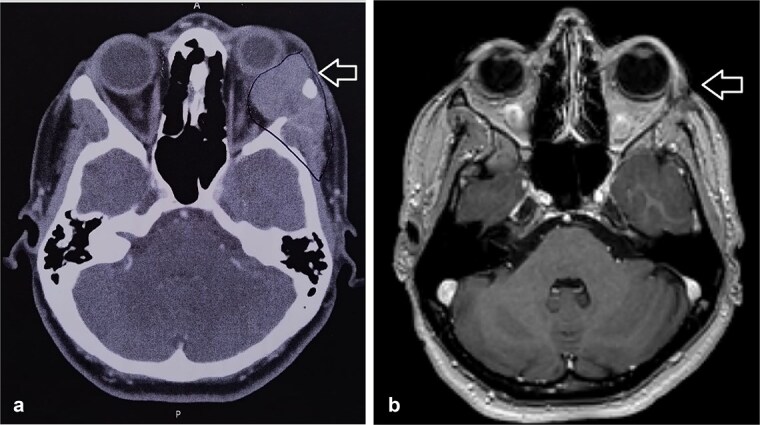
(a) CT scan showed left orbital mass with both solid enhancing and cystic components. The epicenter of the mass appears to be in the left lacrimal gland. (b) MRI postoperative that showed complete tumor excision.
